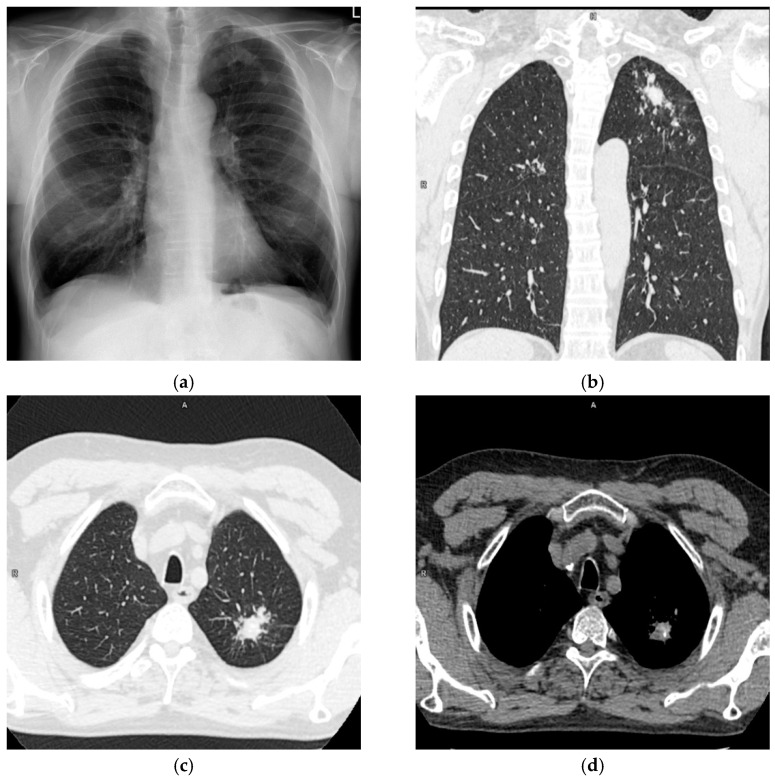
Chest X-ray and CT (a). Chest X-ray, posteroanterior projection. Irregular shape consolidation in the upper zone of the left lung. (b). Chest CT, lung window, coronal image. Various shape and size lung nodules in the left upper lobe. (c) Chest CT, lung window, axial image. The largest lung nodule with spiculated borders is in the left upper lobe. (d) Chest CT, mediastinal window, axial image. Calcifications in lung nodules.
